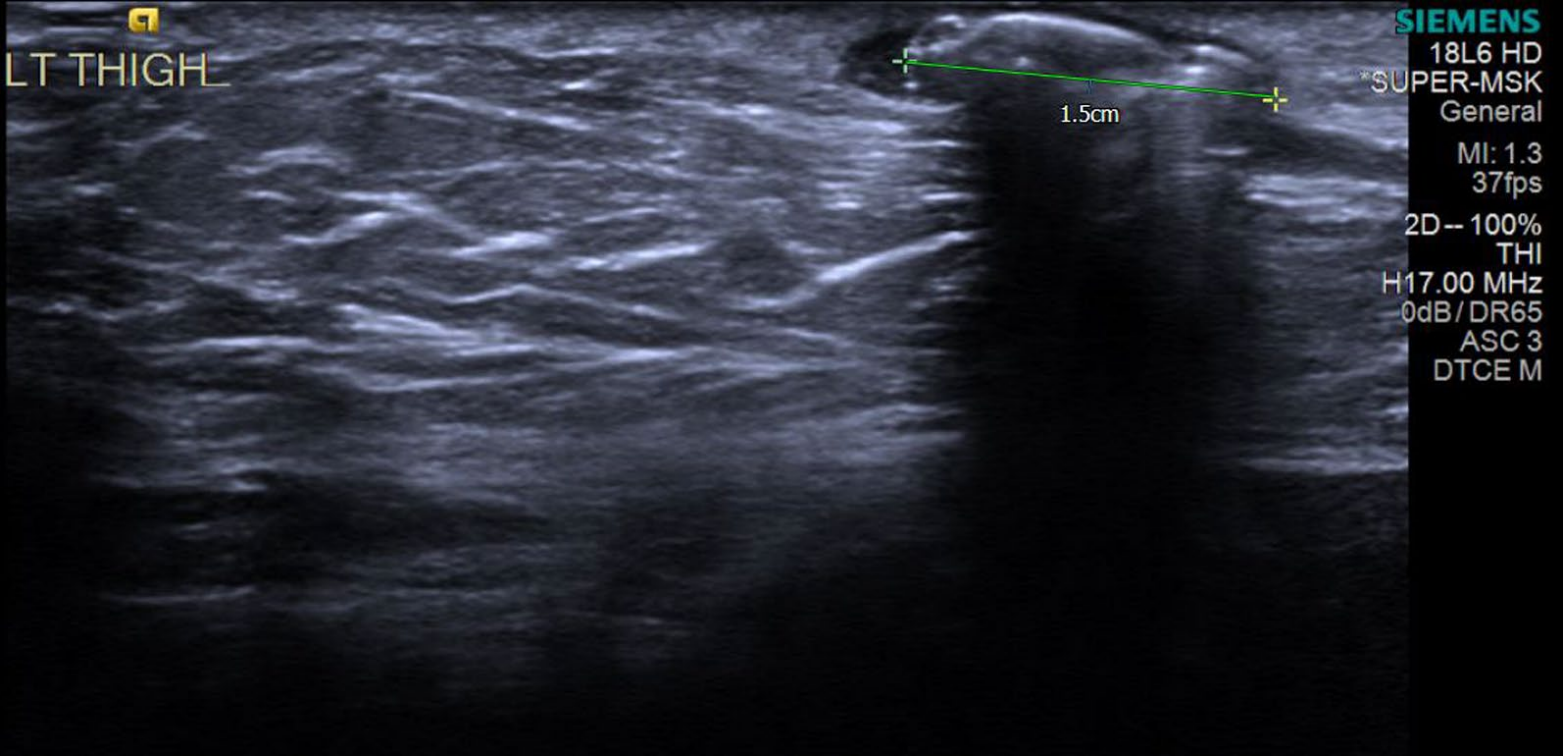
Admission ultrasound image showing foreign body in the subcutaneous tissue of the posteromedial left thigh
